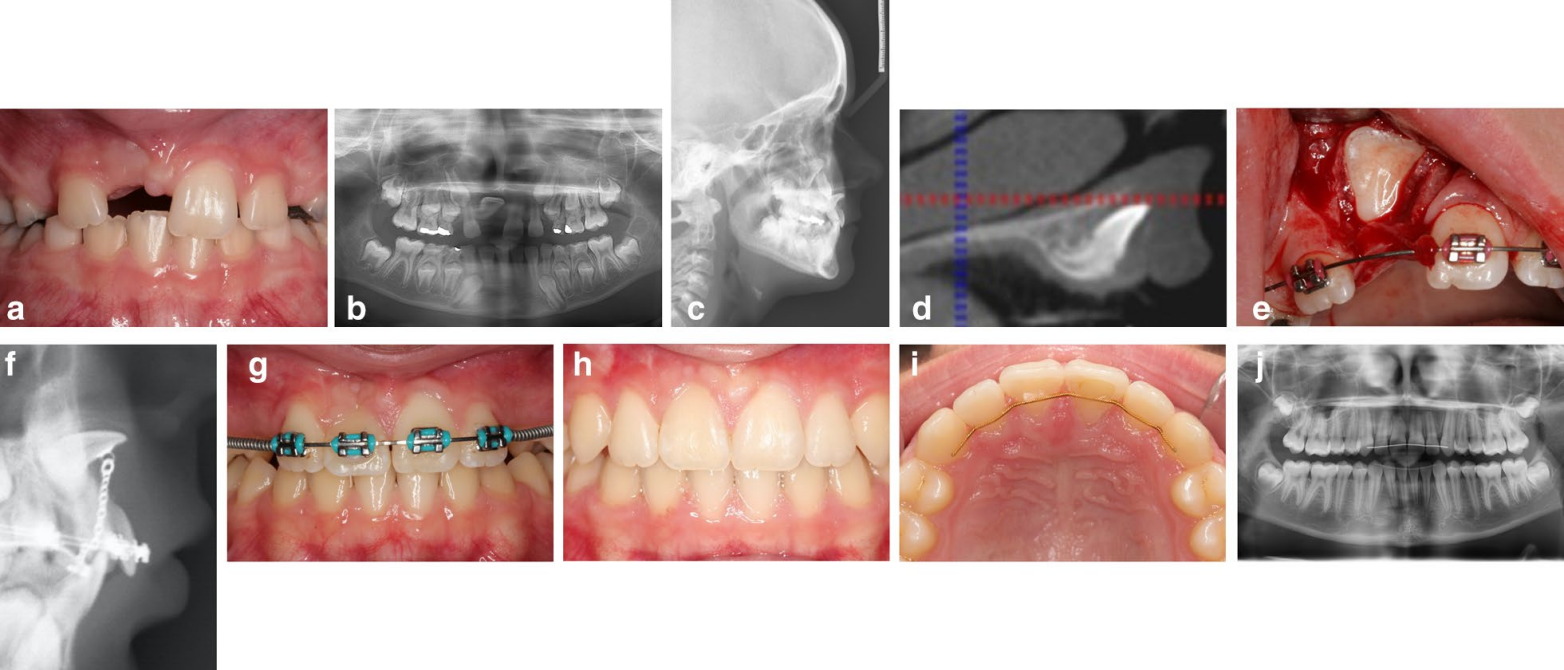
a: A seven-year-old patient with complete eruption of both lateral incisors and left central incisor. b: Panoramic X-ray image of tooth 11 positioned horizontally with apparent root angulation. c: Cephalometric X-ray image of the crown of tooth 11 is inclined in cranial direction. d: Cone-beam computed tomography sagittal view showing root dilaceration is evident. e: Lifting the mucoperiosteal flap and exposing the palatal surface of the crown of tooth 11 prior to bonding of the attachment. f: Cephalometric X-ray image showing the attachment on the palatal surface and chain traction in the palatal direction toward the extension arm of the palatal arch. g: Impacted right central incisor aligned into the dental arch. Attached gingiva is apparent due to the eruption of the tooth through the alveolar ridge crest. The crown torque is insufficient. h: Ideal gingival margins and equal amount of attached gingiva on teeth 11 and 21 at debonding. i: Ideal crown torque of tooth 11 from the occlusal view after debonding. j: Panoramic X-ray after treatment demonstrating the same root lengths on teeth 11 and 21
